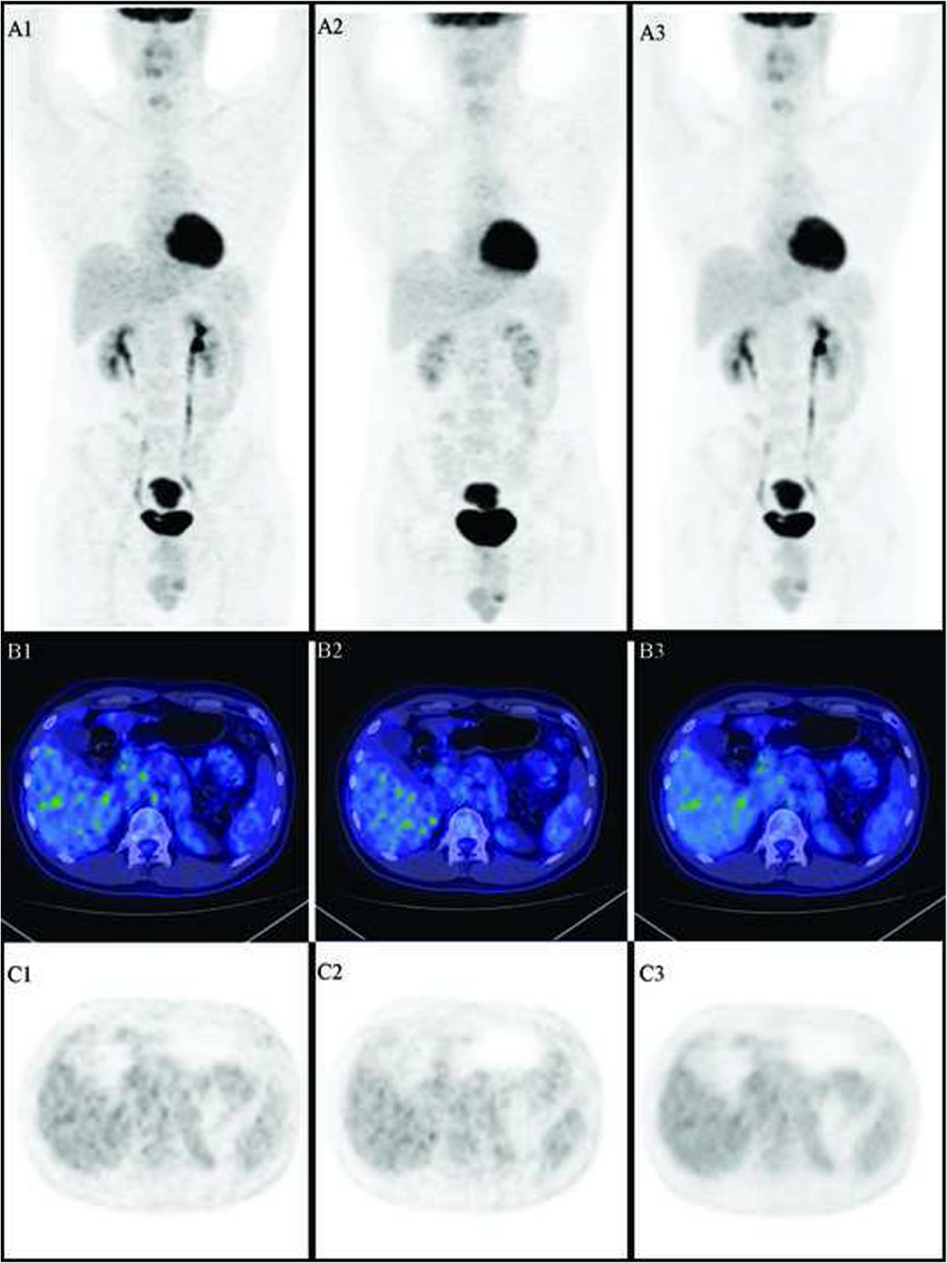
Staging of sigmo-rectal adenocarcinoma (new diagnosis). Patient injected with 66% dose of 18F-FDG. 1. Scan with reduced number of counts (acquisition with standard time and 66% FDG dose). 2. Native scan: scan with standard number of counts (acquisition with increased time). 3. Processed scan, resulting from the study with reduced counts (1) processed by AI SubtlePET^TM^. a Maximum intensity projection (MIP). b Fused transaxial positron emission tomography and computed tomography. c Transaxial positron emission tomography images. All images were visualized with AW server station by setting the same parameters (DFOV 52.0 × 32.2 cm, *M*=4.49 g/ml). Distribution of the radiotracer, in the MIP and in the transaxial images at the level of the liver, shows a good image quality both in native and in the processed study
